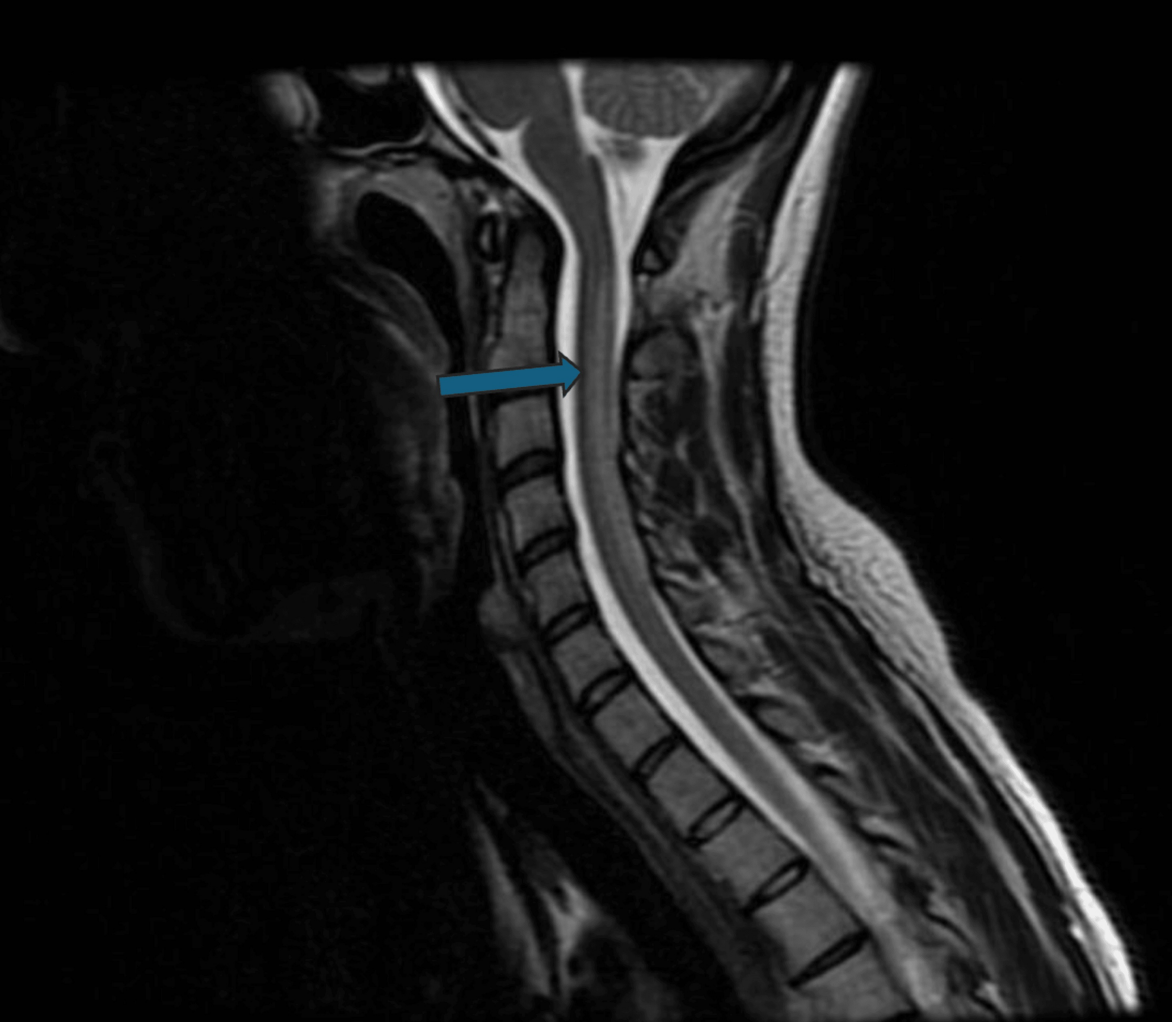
MRI of the cervical spinal cord showing demyelination. This T2-weighted MRI scan shows hyperintensity in the dorsal aspect of the cervical spinal cord. The hyperintensity is most prominent at the C1 to C5 levels, which suggests demyelination or inflammation in this region (blue arrow).
